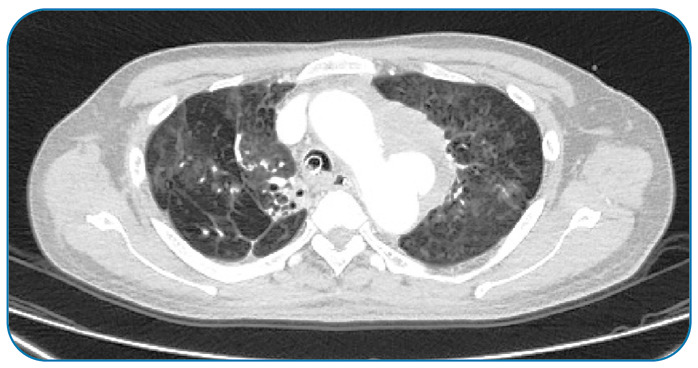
Computed tomography angiogram showing a ruptured aortic arch saccular aneurysm (arrow).
